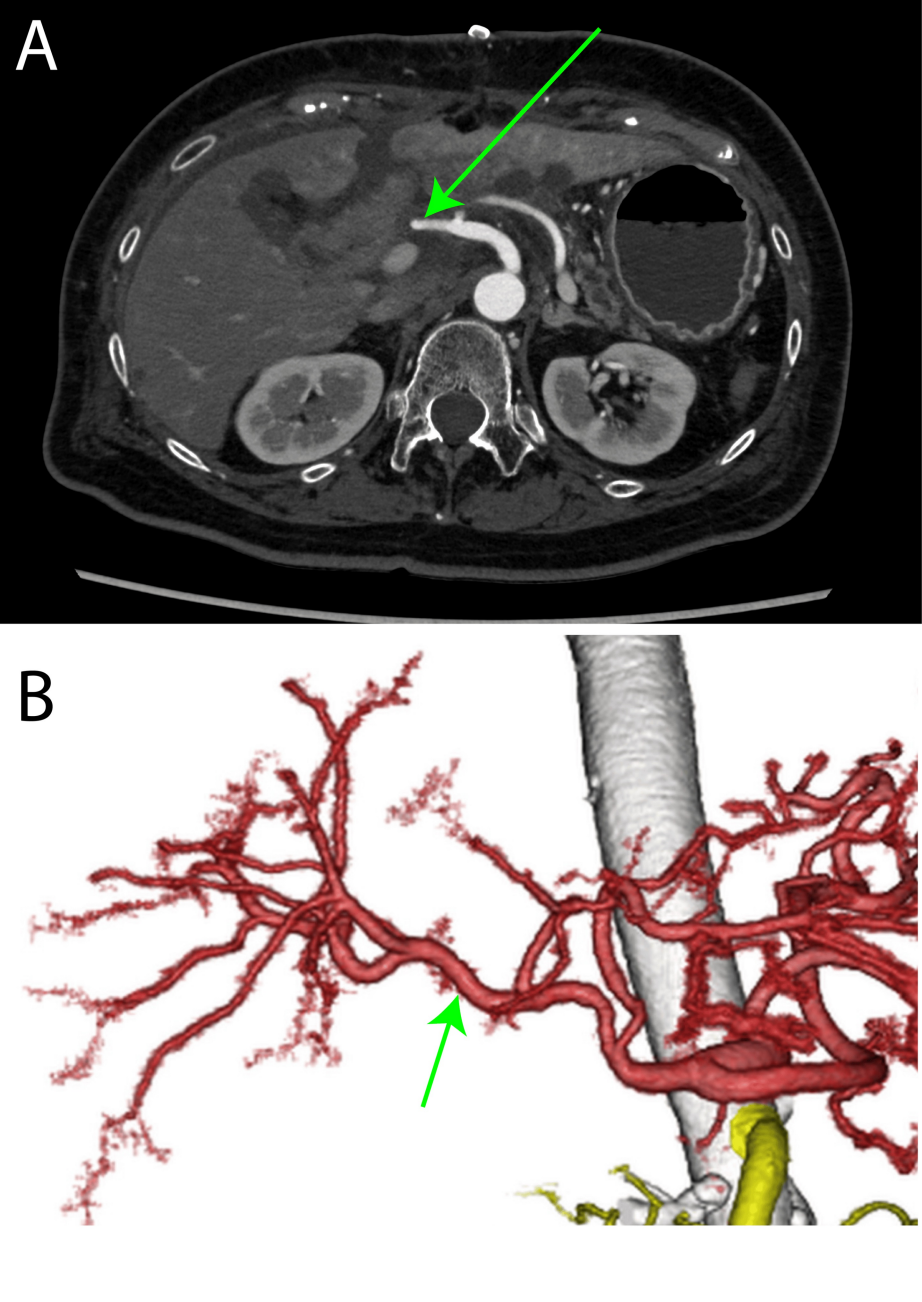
Postoperative CT (A) Contrast-enhanced CT showed the anastomosis site with common hepatic artery and proper hepatic artery (green arrow); (B) Volume-rendered (VR) image demonstrating the anastomosis site with common hepatic artery and proper hepatic artery (green arrow).
